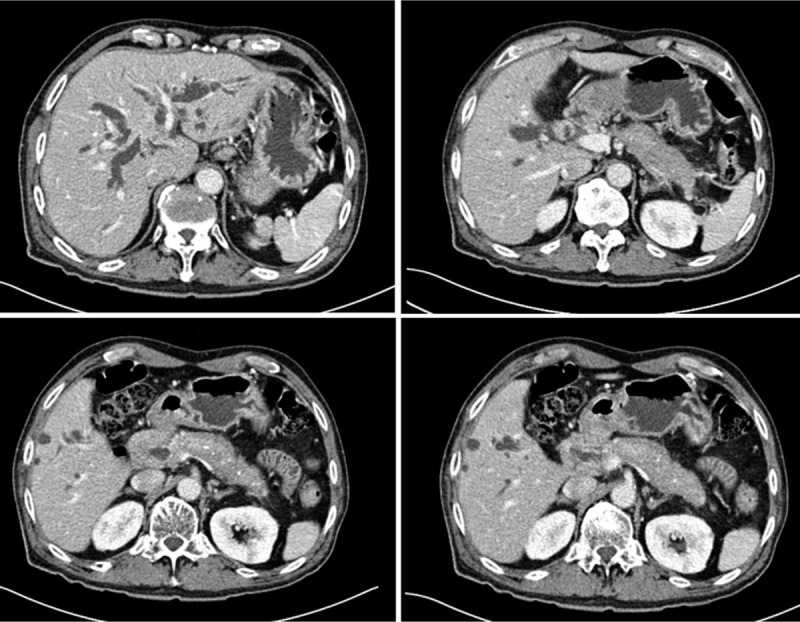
Computed tomography showed ductal wall swelling and enhancement from both intrahepatic duct confluence to the common bile duct, upper biliary dilatation. In addition, the pancreas was swollen and showed low attenuation at its periphery.
